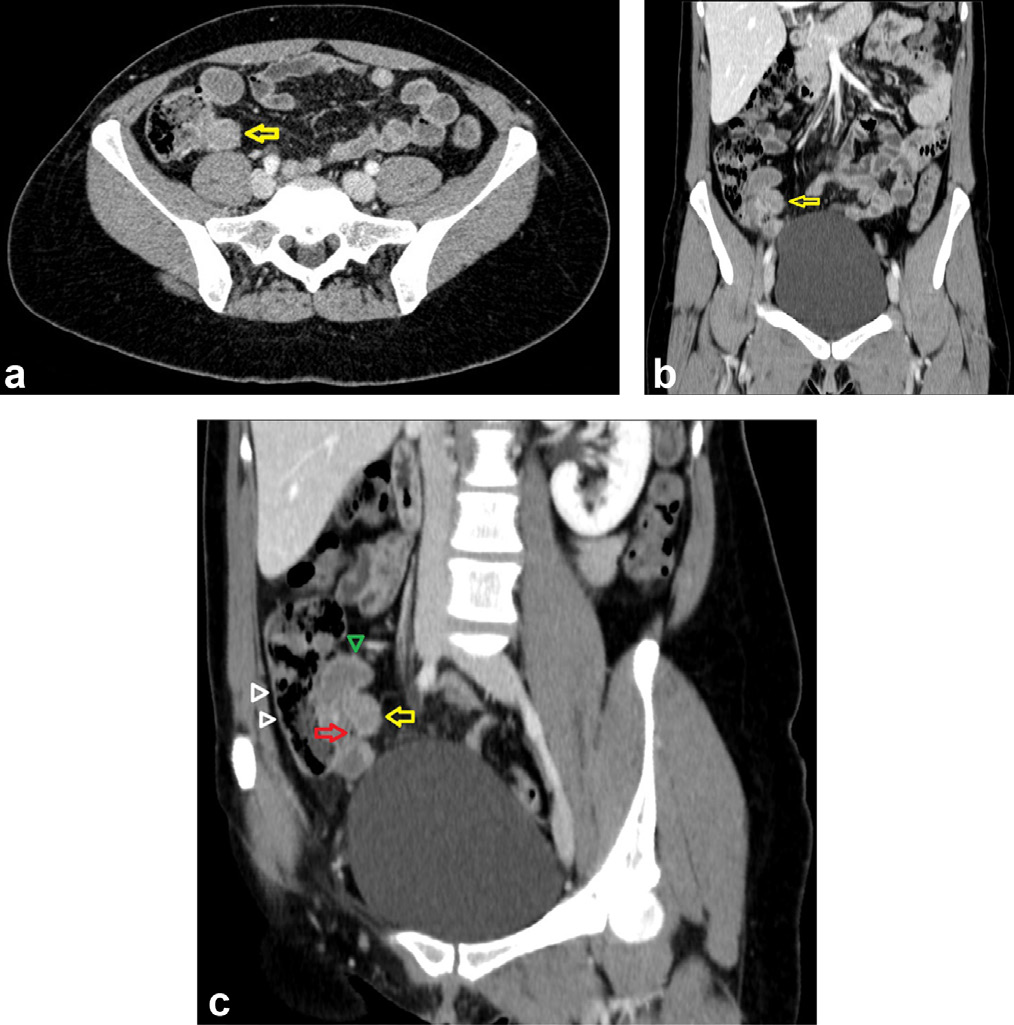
(a, b) CT examination depicted a non-specific focal mass (yellow arrow) in the distal third of the appendix without any evidence of inflammation. (c) Coronal modified view better depicts the relation of the appendiceal mass (yellow arrow) with the ileum (green arrowhead), appendix (red arrow pinpointing the air bubble in its lumen), and the cecum (white arrowheads).
